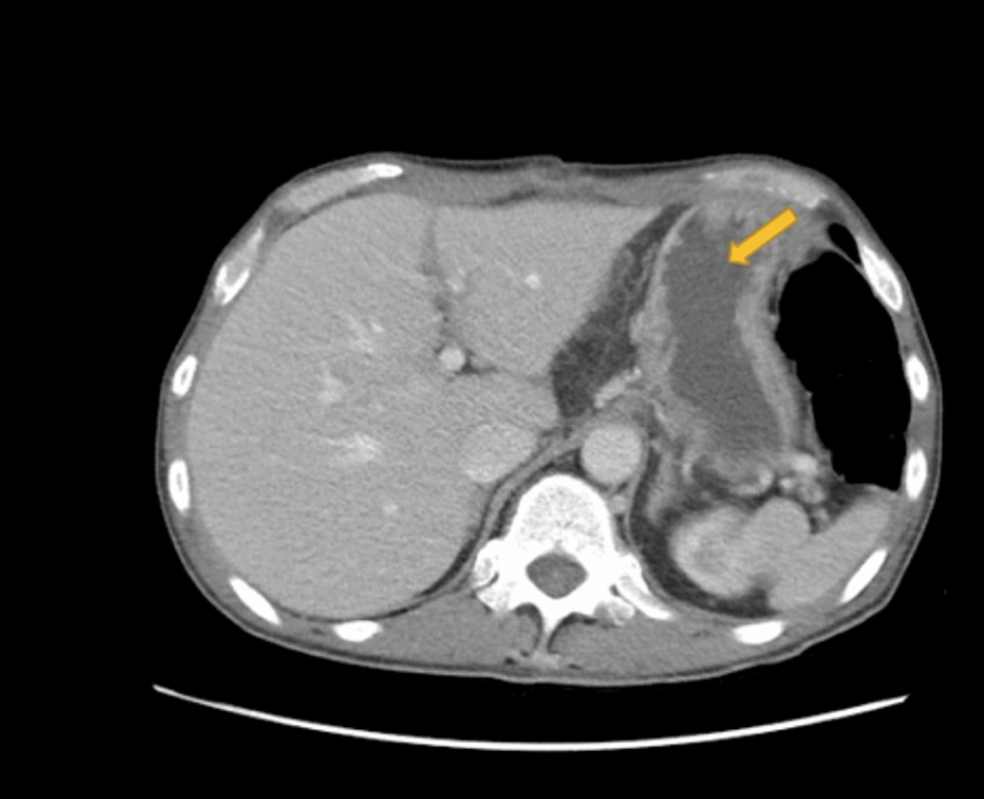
CT of abdomen and pelvis with contrast showing thickening involving the descending duodenum (yellow arrow)
